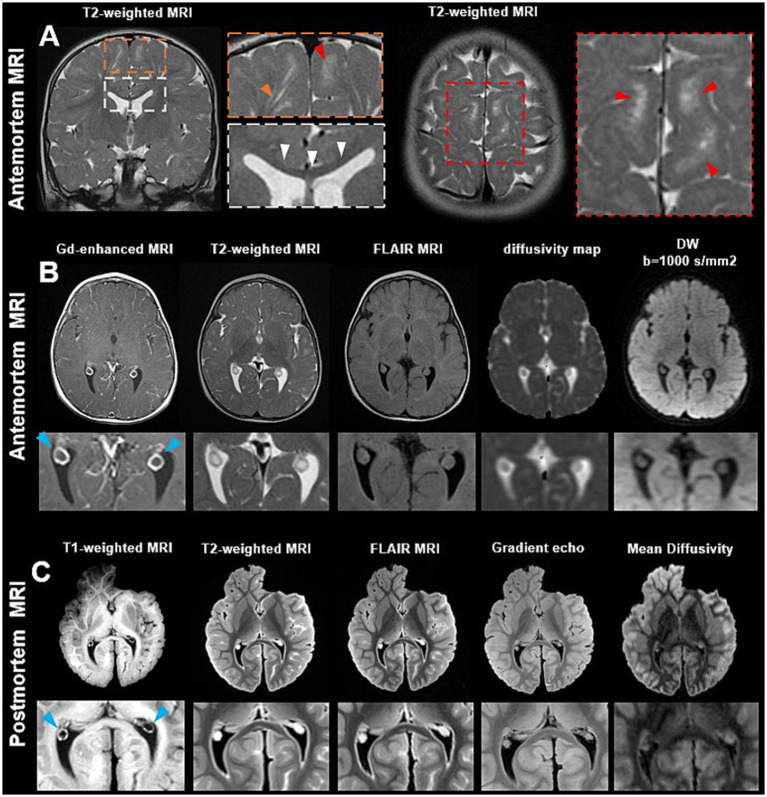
Antemortem clinical MRI demonstrates thinning of the corpus callosum and white matter hyperintensities, including enlarged perivascular spaces (orange arrowhead) and small areas of clustered tiny perivascular spaces (red arrowheads) on T2-weighted images (A). Antemortem MRI (B) including gadolinium-enhanced T1-weighted, T2-weighted, and FLAIR images demonstrates symmetric cystic changes of the choroid plexus (ChP) with T2 hyperintensity and incomplete FLAIR suppression, indicating a non-CSF component. In addition, diffusivity/ADC maps and diffusion-weighted imaging (DWI, b = 1,000 s/mm^2^) show relatively low ADC values and increased DWI signal compared with CSF, consistent with restricted diffusion. Postmortem MRI (C) including T1-weighted, T2-weighted, FLAIR, gradient-echo, and mean diffusivity sequences, confirms the bilateral cystic morphology of the ChP. Notably, cyst size showed minimal change when comparing the antemortem MRI acquired at 2 years of age with the postmortem MRI obtained at 12 years of age.
